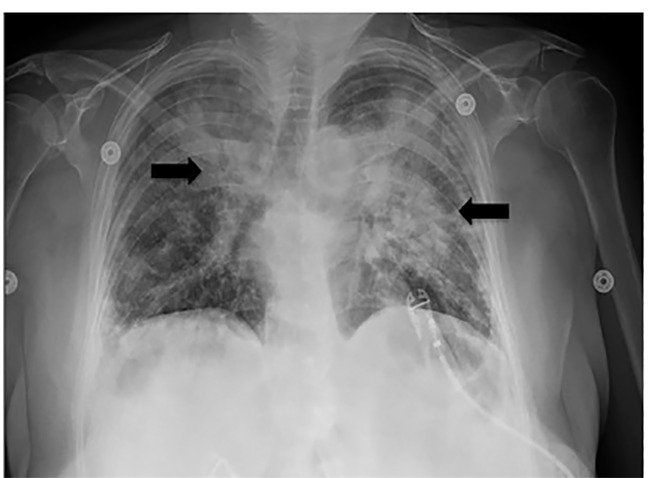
Radiograph of this patient with coronavirus disease 2019 demonstrates dense patchy airspace disease bilaterally (arrows).
